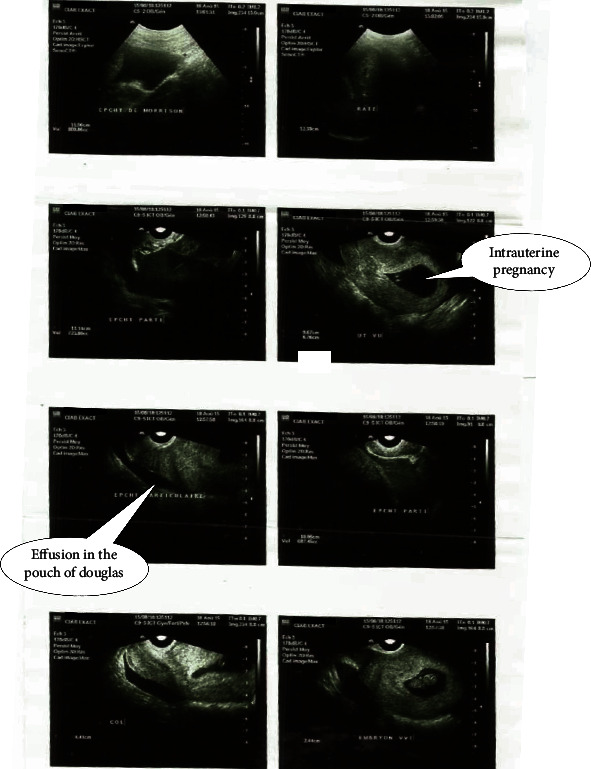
Ultrasound images showing intrauterine pregnancy and effusion in the Douglas pouch.
